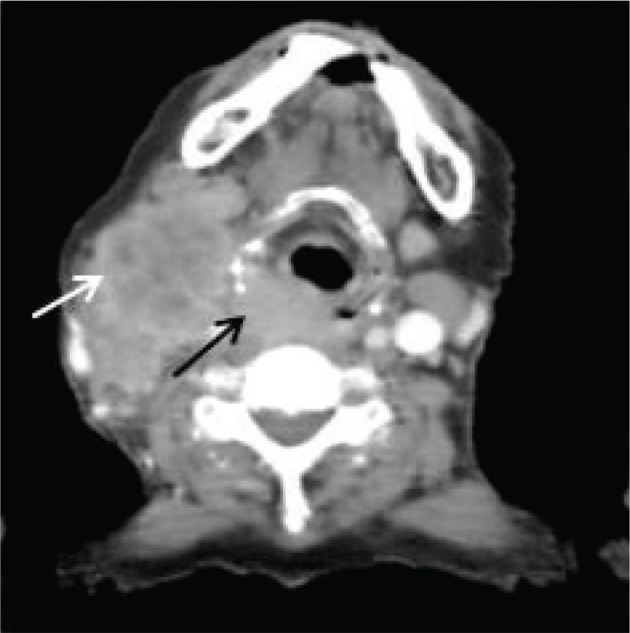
Contrast-enhanced CT scan image through the region of hypopharynx showing a soft tissue density enhancing mass, primarily involving the right side obliterating the right piriform sinus (black arrow). It was extending inferiorly to involve the proximal esophagus. There is also associated right deep cervical confluent nodal mass (white arrow). CT, computed tomography.
